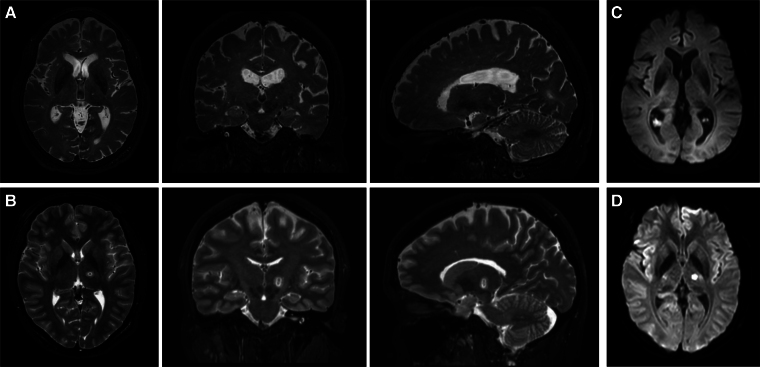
MR imaging results for Case #9 and Case #12. (A and B): The axial, coronal and sagittal views of the T2 weighted MR images are shown. No lesion was observed in the post-procedure MR for patient Case #9 (A). A typical MRgHIFU T2 weighted lesion is shown in (B) for comparison. (C and D): Axial view of diffusion-weighted imaging (DWI). No diffusion restriction was observed in Case #9 (C), in contrast to Case #12 (D). MR, magnetic resonance.
